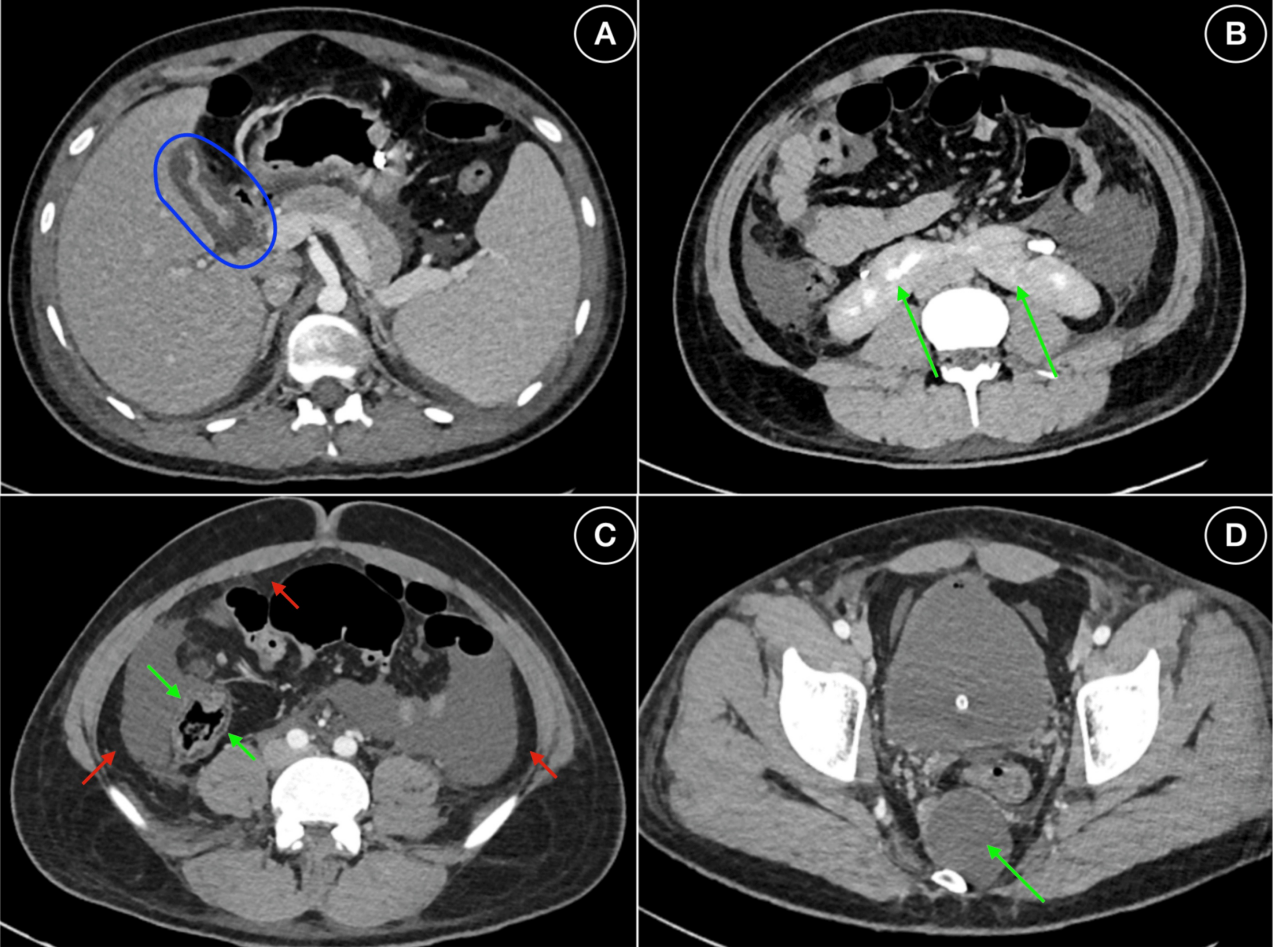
Contrast-enhanced computed tomography of the whole abdomen. (A) Contracted gallbladder with surrounding pericholecystic fluid suggestive of acalculous cholecystitis (blue oval). (B) Lower poles of kidneys fused in the midline by a membranous isthmus forming a parenchymal bridge at the L3 vertebral level, consistent with horseshoe kidneys (green arrows). (C) Edematous thickening of the ascending colon, indicating inflammatory/infective pathology (green arrows), with associated ascites visible (red arrows). (D) A well-defined, rounded, hypodense benign lesion measuring 4.4 × 4.2 × 4.3 cm in the sacral region posterior to the rectum, suggestive of a tail-gut cyst (green arrow).
